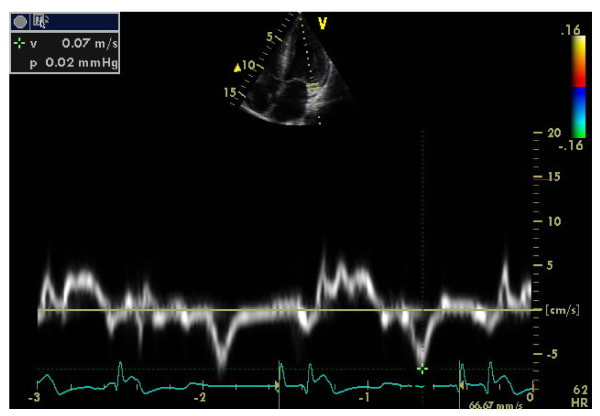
Tissue Doppler of the mitral annulus: near normal systolic function. Courtesy: Pr Albert A. HAGEGE, University René Descartes, Paris, France.
